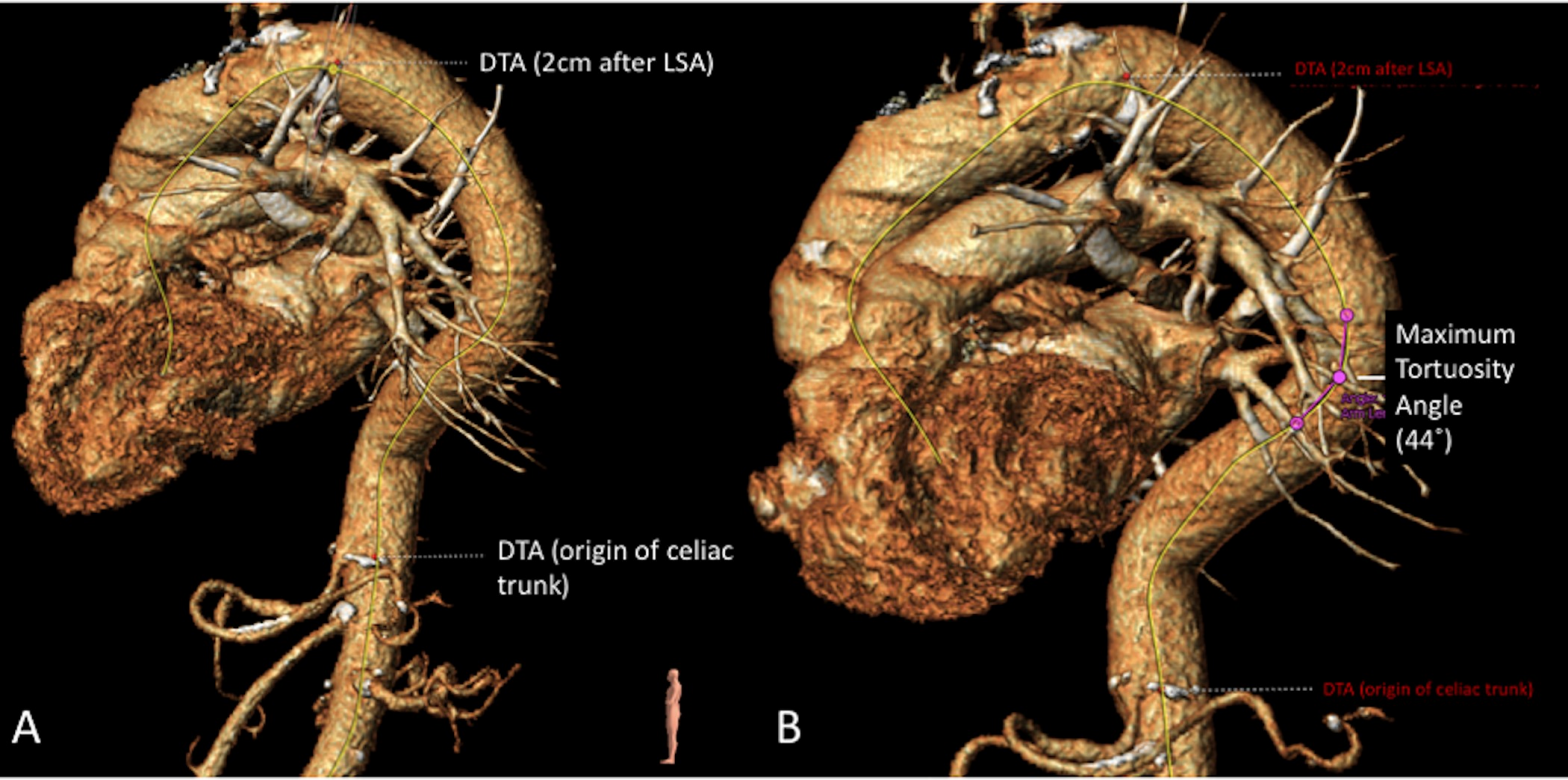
CT scan measurements performed in 3Mensio software. Two images of measurements in 3Mensio in the DTA; A: Center lumen line created for zone 4 (yellow line), with the start of the DTA 2 cm after the LSA up to the celiac trunk; B: *Maximum tortuosity angle* with two line elements that are defined by three control points over the centerline to measure the tortuosity in degrees.
